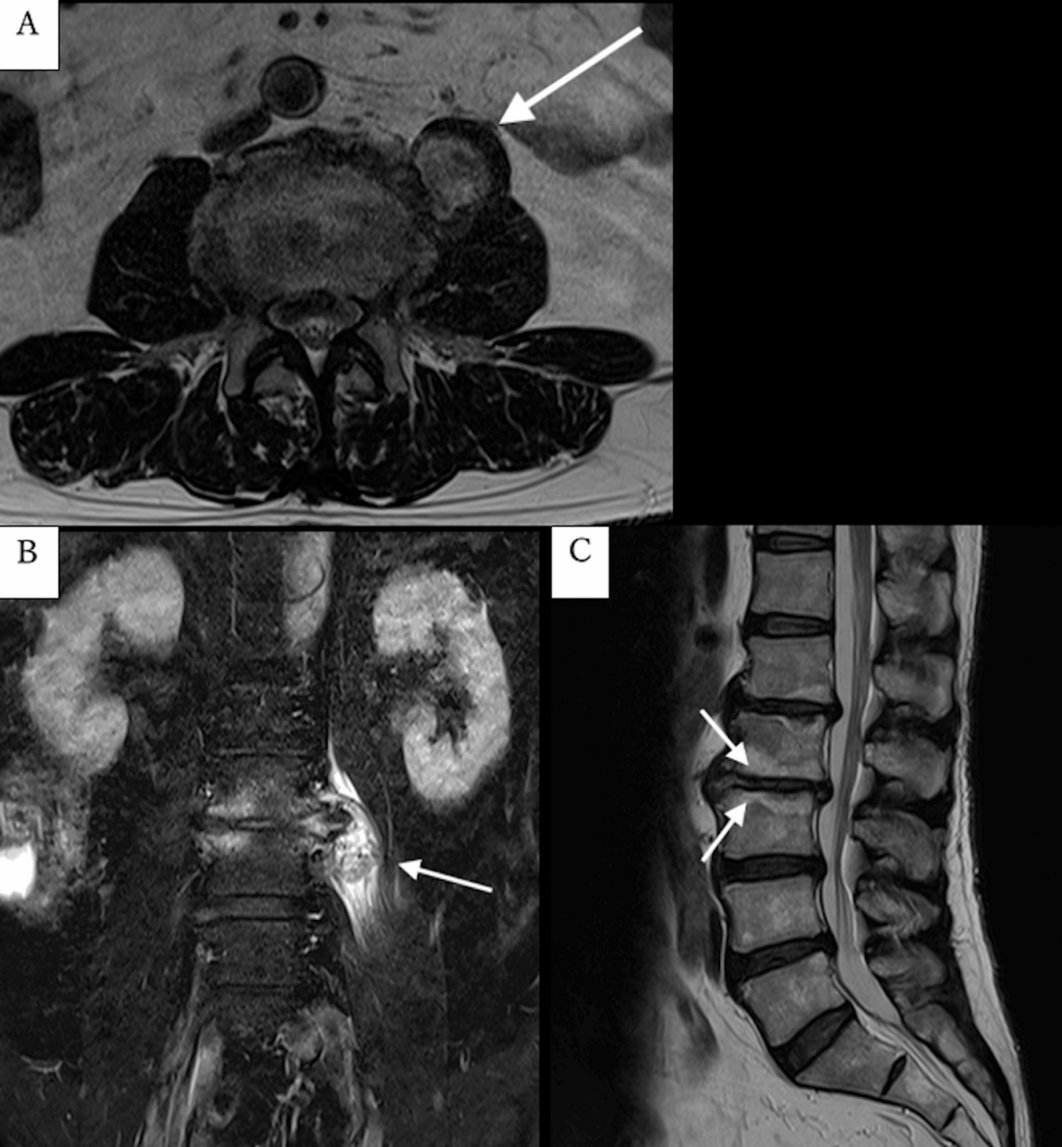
Magnetic resonance imaging findings (A) The left psoas mass shows high signal intensity with mixed low signal on T2-weighted images at the L2/3 level (arrow). (B) The surrounding muscle shows a high signal intensity on short tau inversion recovery (STIR) (arrow). The L2-3 vertebrae adjacent to the mass showed disc bulging, osteophyte formation, and endplate edema. (C) The preserved endplate contour on T1-weighted images at the L2/3 level (arrows).
